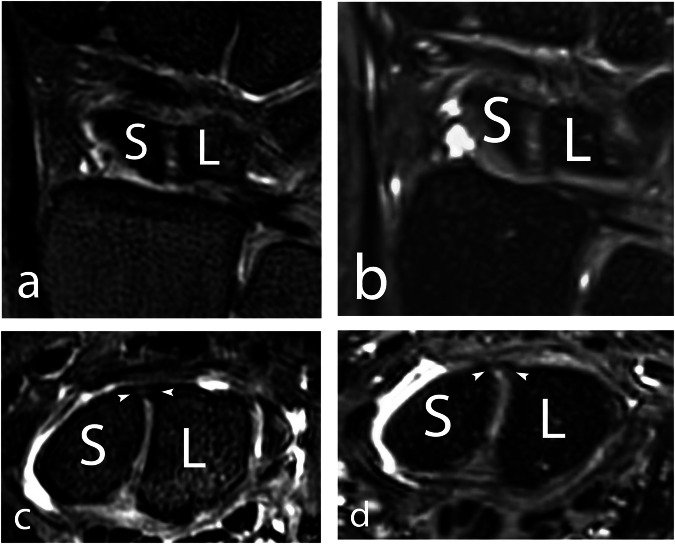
A 44-year-old male with an intact dorsal component of the SLL on 7-T and 3-T MRI. The dorsal component of the SLL (arrowheads) can be clearly delineated on coronal and axial reformations at (a, c) 7-T and (b, d) 3-T 3D PD-weighted MRI. SLL, scapholunate ligament; T, Tesla; S, scaphoid; L, lunate
